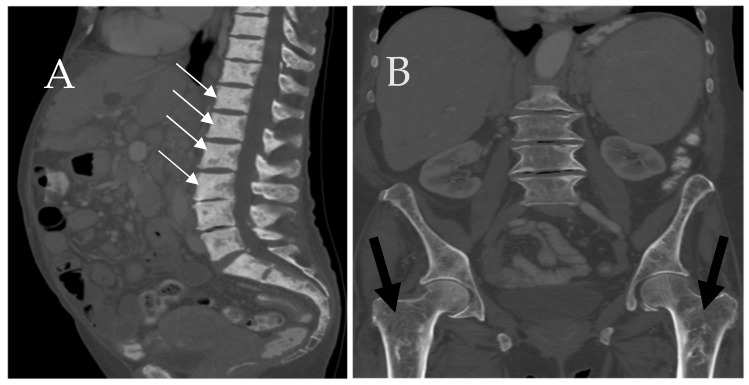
A 67-year-old female patient presenting with back pain. (A) Sagittal CT of the spine shows diffuse sclerosis of the axial skleton (white arrows). (B) Coronal CT shows a diffuse loss of normal bone trabeculations in bilalateral proximal femurs secondary to mast cell infiltrations (black arrows).
